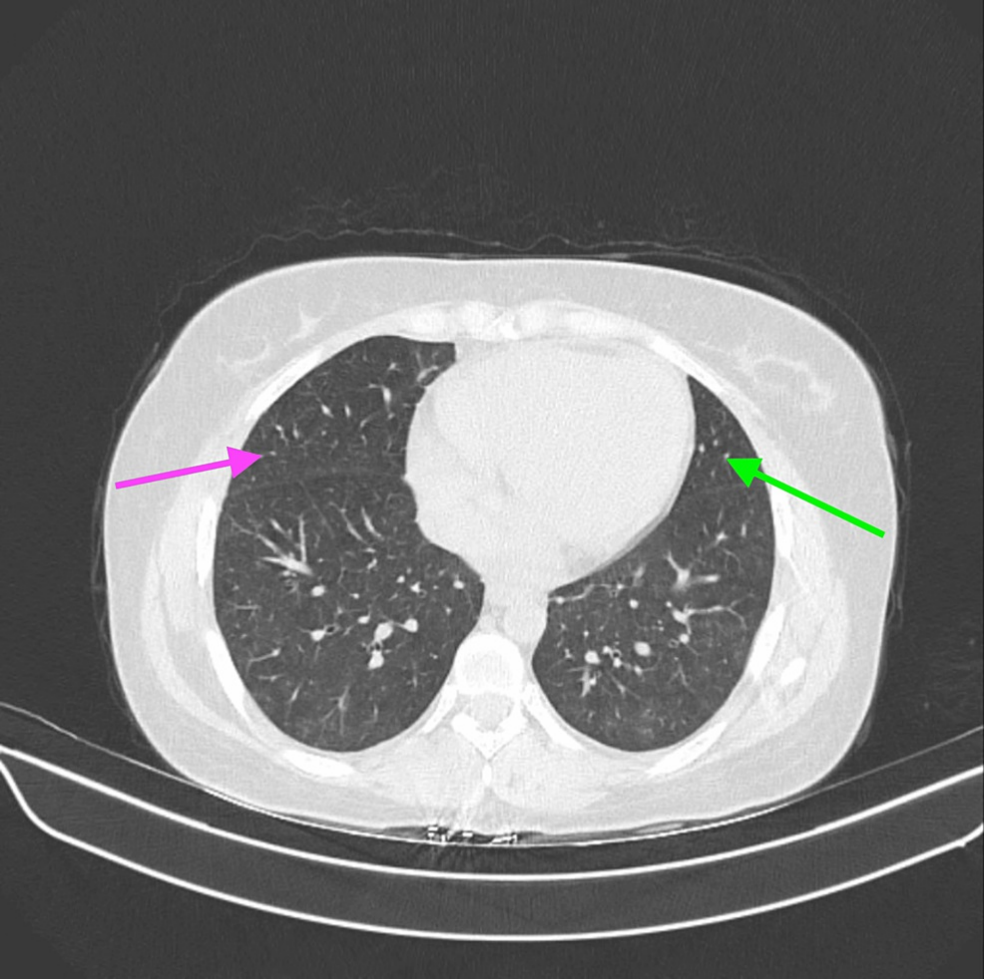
High-resolution computed tomography (HRCT) thorax showing a resolution of ground-glass opacities in the right lung parenchyma (pink arrow) and left lung parenchyma (green arrow)
